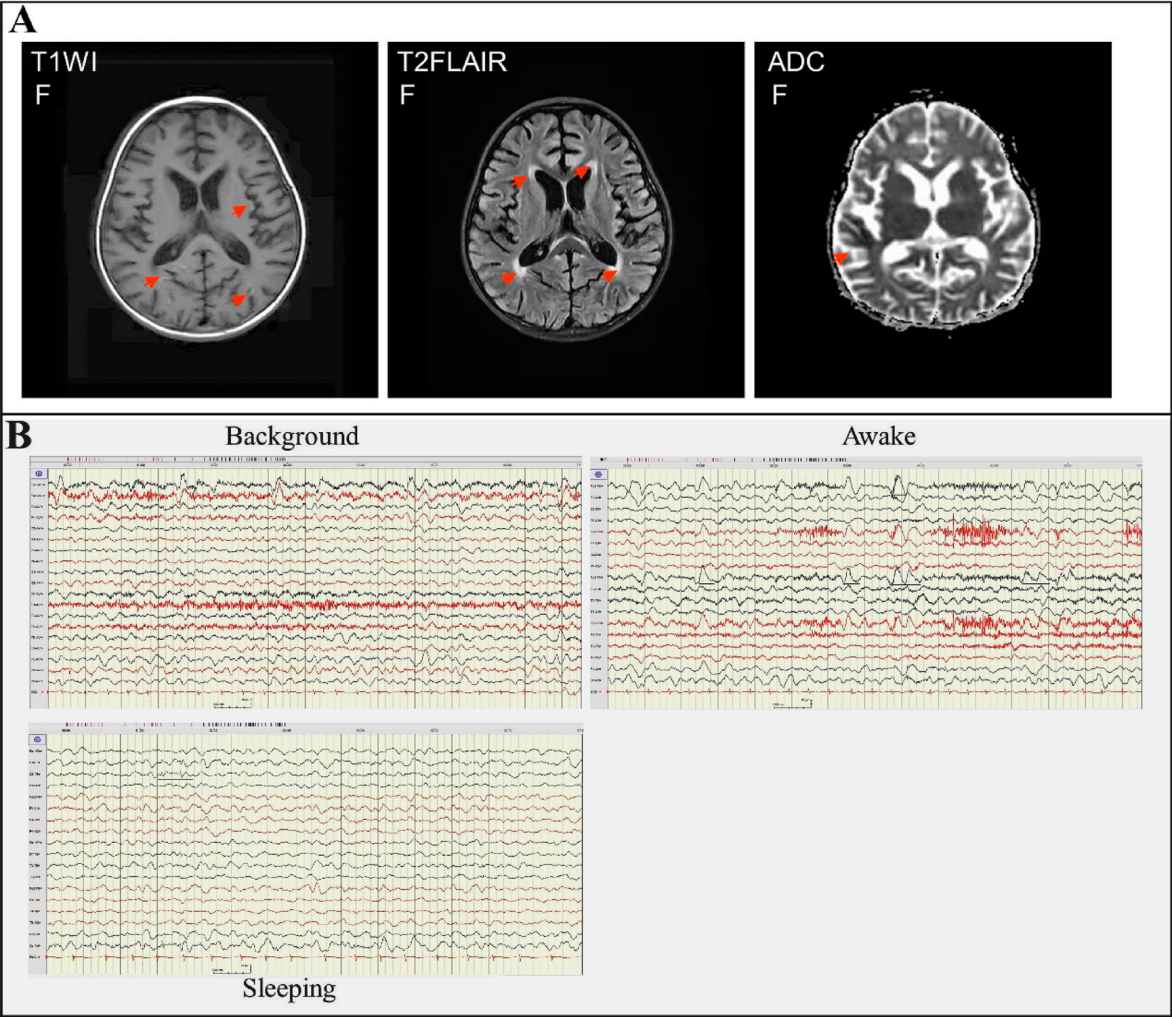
Brain imaging and EEG on day 28 of the illness. Panel A: MRI of the brain indicated abnormal signals on DWI, ADC, and T2-FLAIR images (marked with an arrow). DWI showed lamellar necrosis of the cerebral cortex; T2-FLAIR indicated cerebral sulci, bilateral lateral fissure cisterns, and lateral ventricle enlargement. Panel B: EEG presented the relative enhanced θ and δ activities with the continuous asymmetrical low waves manifested spike, sharp, or slow wave complex (black line marked).
